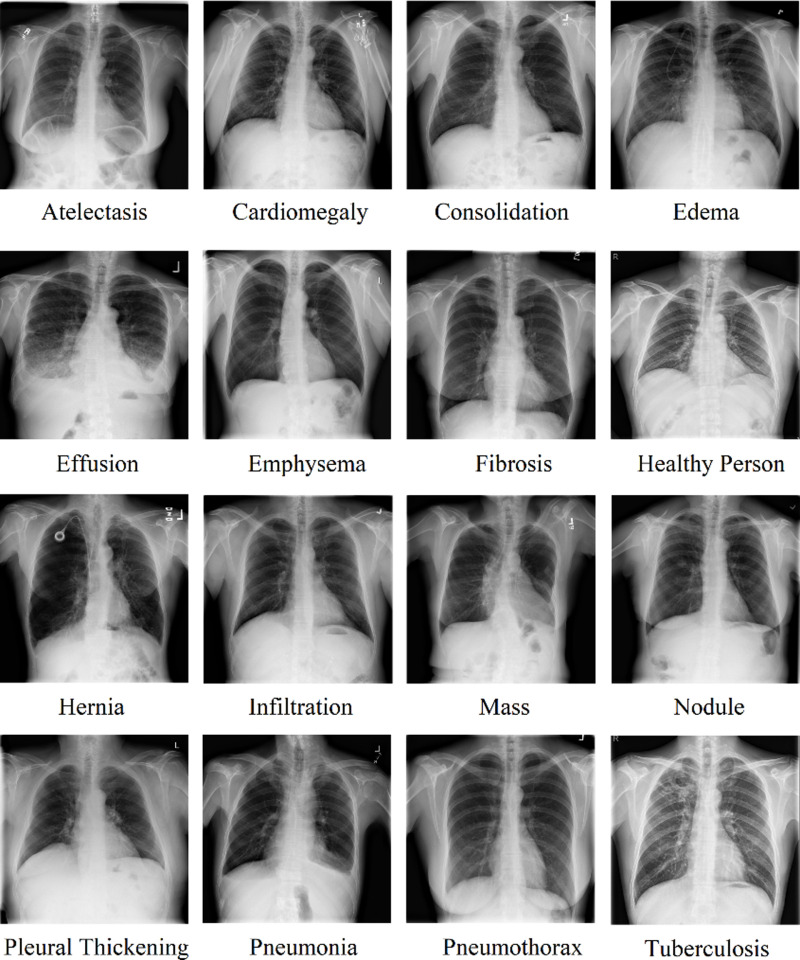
Representative chest radiographs of all classes.
